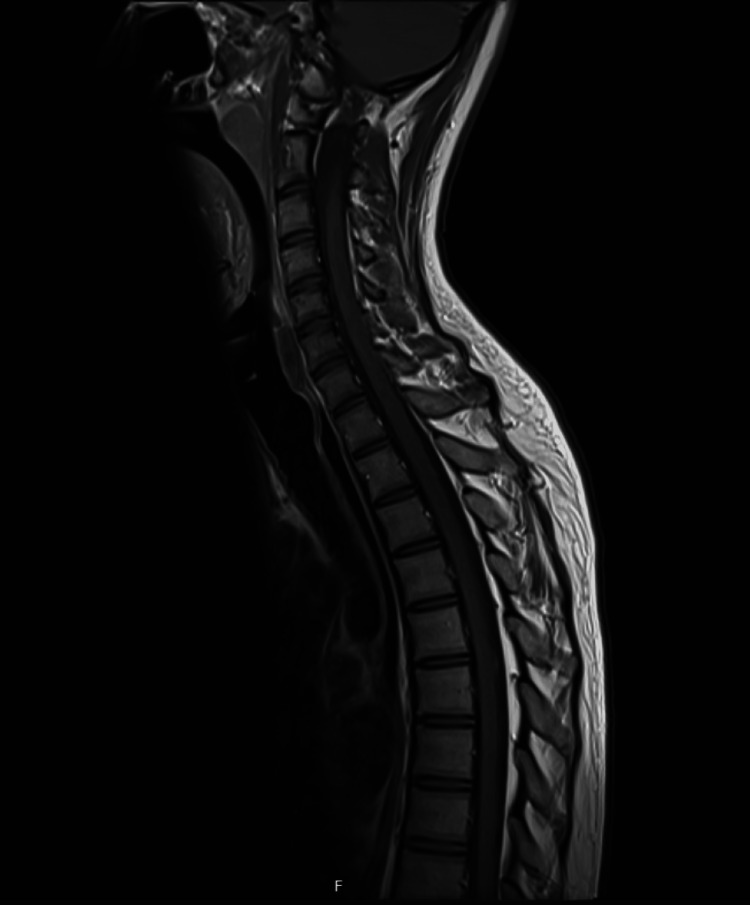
Normal MRI of the spine.
